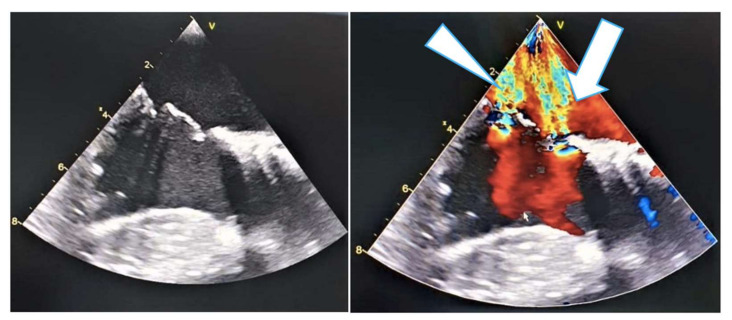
Intraoperative transesophageal echocardiographic evaluation of severe mitral regurgitation. Two regurgitation jets can be identified, one transvalvular (arrowhead) and a second through a perforation in the anterior mitral leaflet (arrow).
